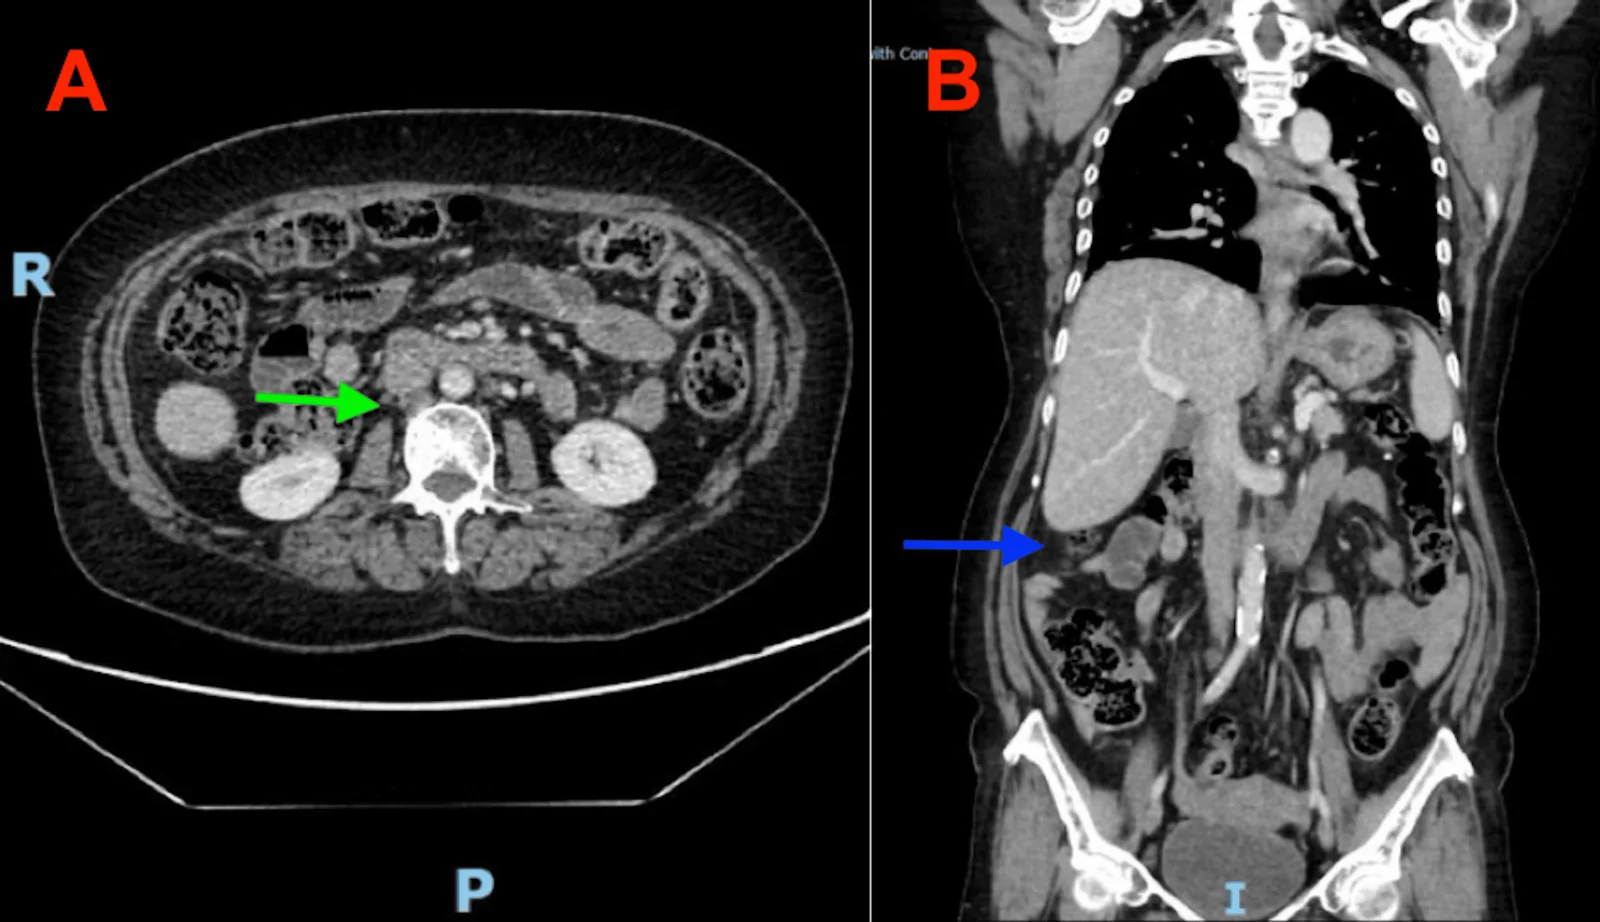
CT Chest, abdomen and pelvis with contrast (07/2023) - transverse (A) and coronal (B) sections, showing marked improvement in the retroperitoneal lymphadenopathy (green arrow) and complete resolution of ascites (blue arrow)
